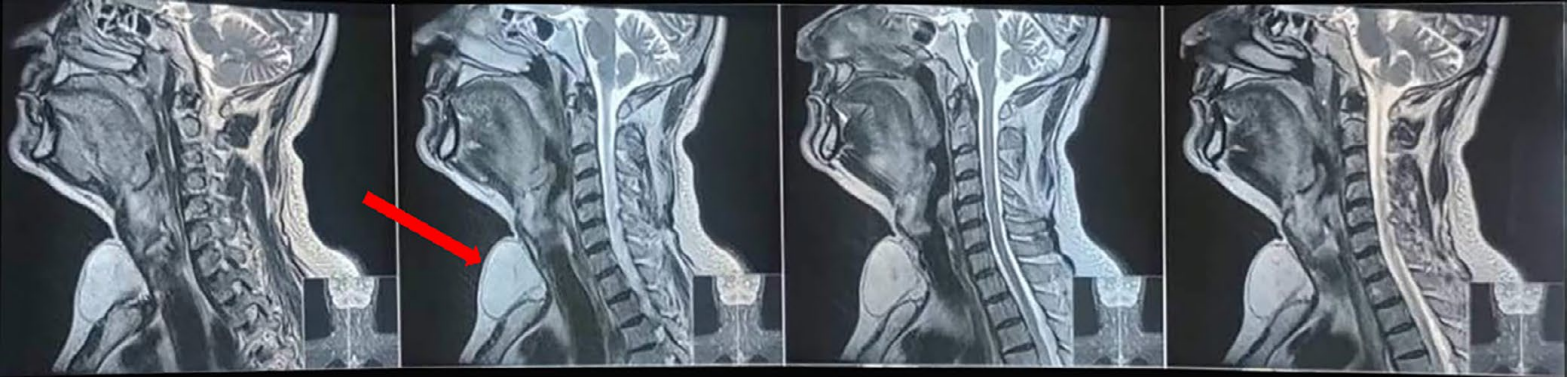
MRI (Sagittal section) neck image of the patient.
